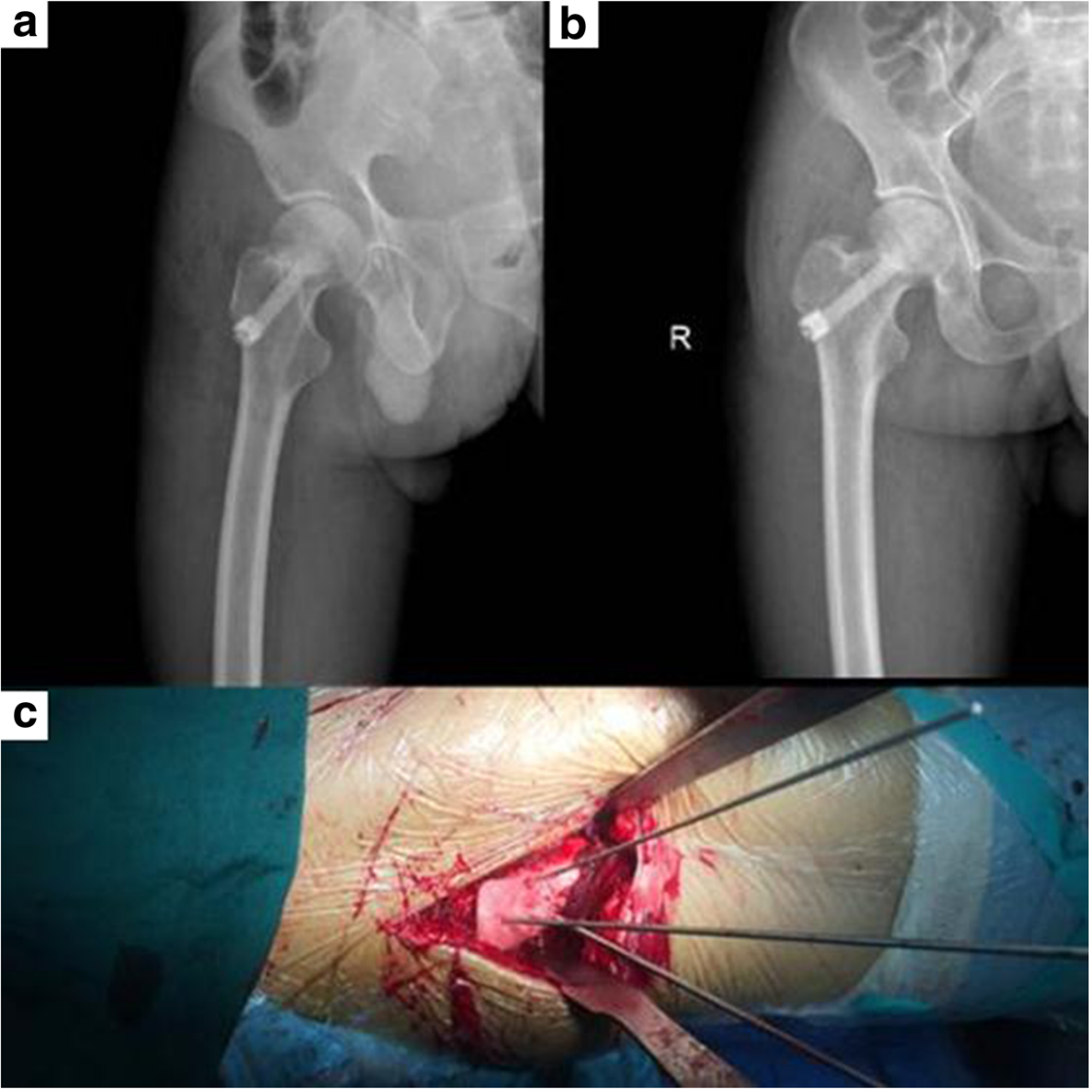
a The post-operative lateral X-ray films. b The post-operative anterior-posterior X-ray films, which showed the good position of core decompression with porous bioceramics rod. c The guide pin path was established under the assistance of individualized 3D guide plate during the operation
